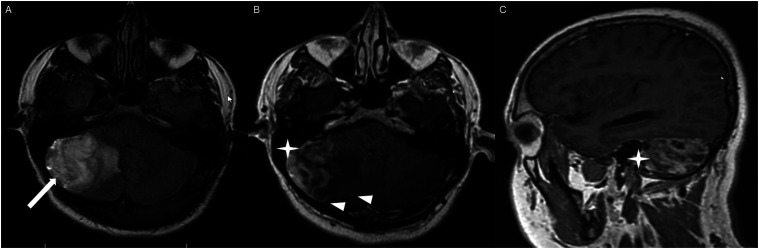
Axial FLAIR (A), axial post-contrast T1 weighted image (B), sagittal post-contrast T1 weighted image (C) show a FLAIR hyperintense mass (A) in the lateral aspect of the right cerebellum (A, arrow), demonstrating heterogeneous and gyriform post-contrast enhancement (B-C, star). There is perilesional spiculated, finger-like enhancement (B, arrowhead).
